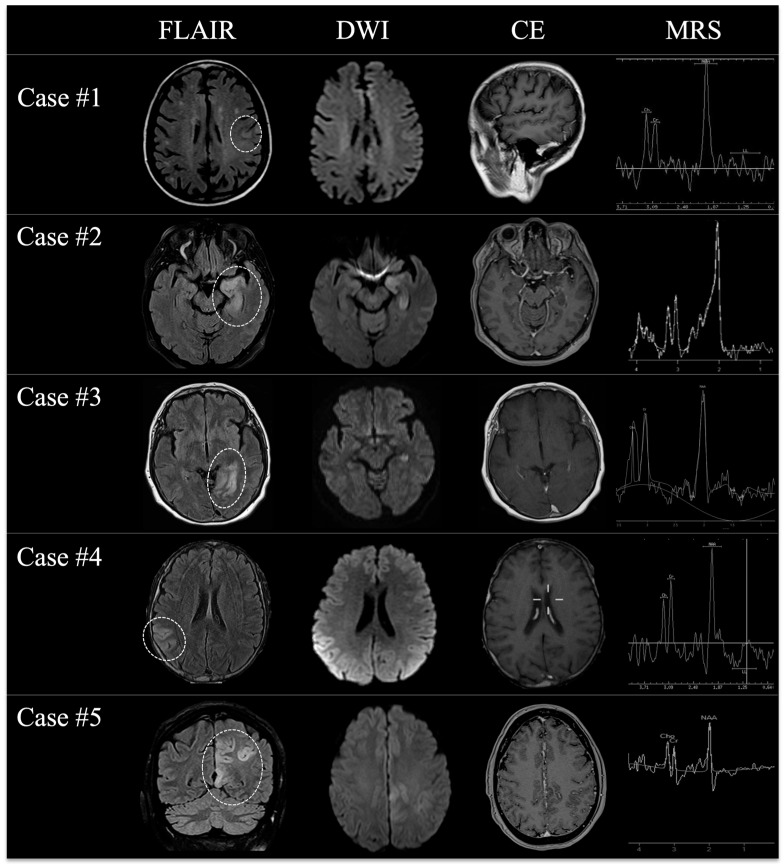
A panel showing the most relevant MRI changes (*dotted lines*) of all patients at the first time-point MRI examination on FLAIR (first column), DWI (second column), contrast-enhanced T1w (third column), and MRS (fourth column) once the initial suspicion of AE was raised.
